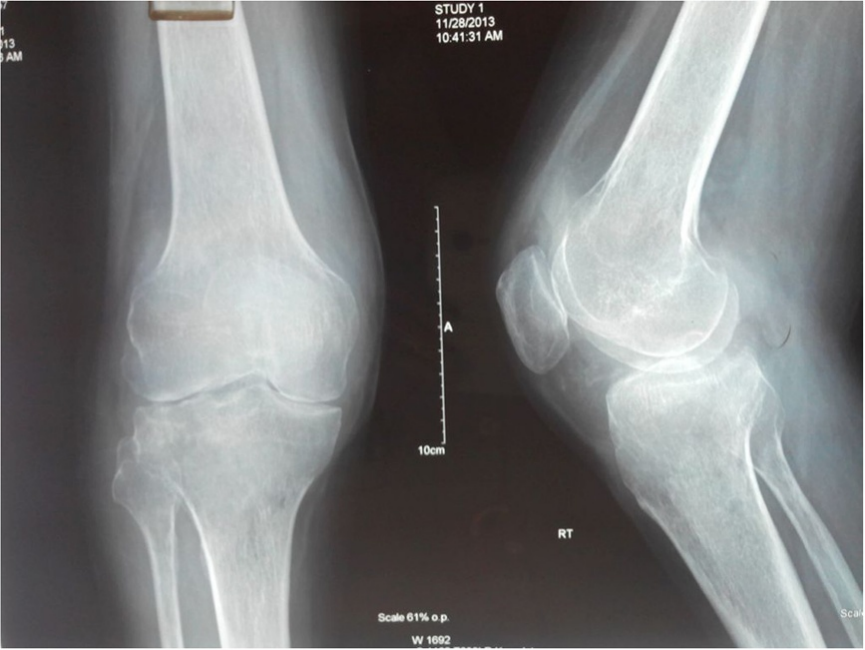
Plain radiograph of right knee. Plain radiograph of the right knee demonstrated mild peri-articular osteopenia with global reduction in joint space along with soft tissue density.
